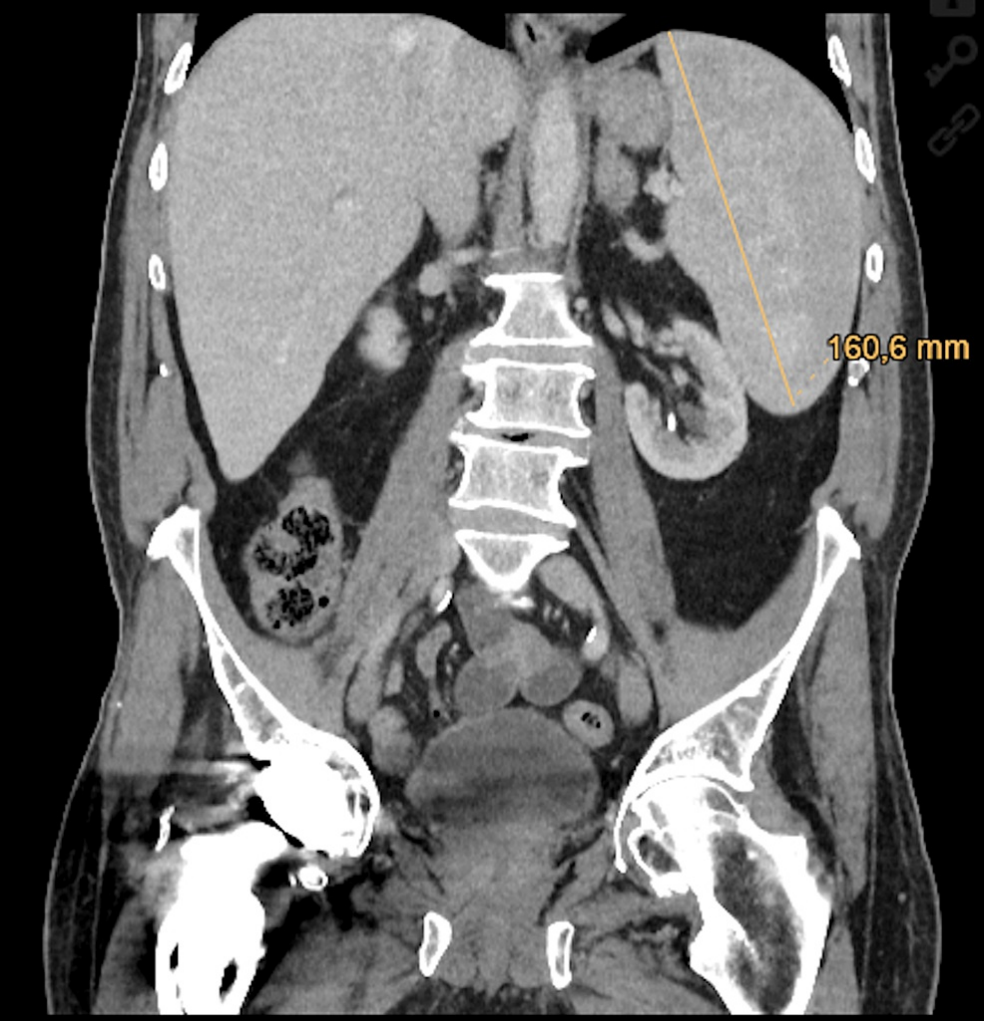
Computed tomography of the chest and abdomen with splenomegaly
